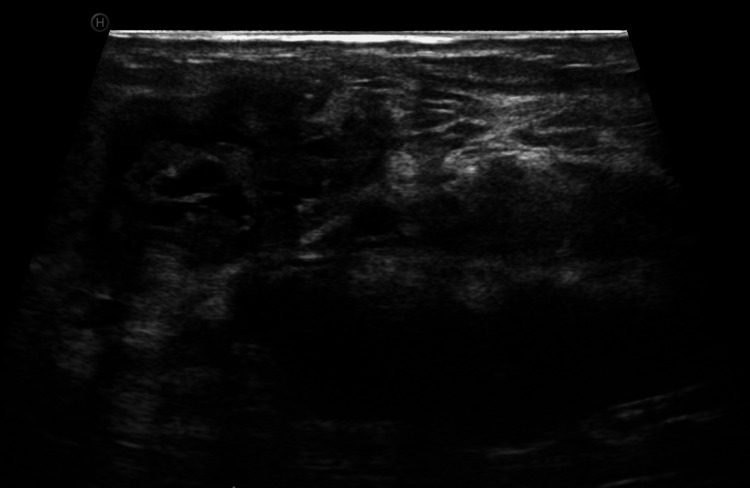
Ultrasound findings Gray-scale ultrasound image demonstrating multiple oval, well-circumscribed nodules with heterogeneous echogenicity, containing cystic components
